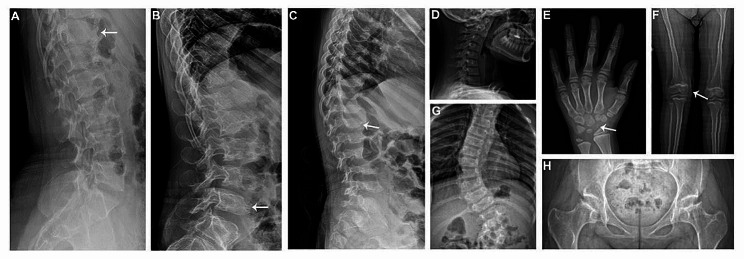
Radiographic features in mild patients with MPS IVA. Lateral lumbar spine radiographs showed irregular vertebral bodies with “tongue-shaped” deformity in Pt1 (A), anterior beaking of the vertebral bodies in Pt8 (B) and anterior sharpening of vertebral bodies in Pt9 (C). Radiographs revealed kyphotic cervical curve in Pt6 (D), dysplastic carpal bones (E) and right genu valgum (F) in Pt5, scoliosis in Pt11 (G) and acetabular and femoral head dysplasia in Pt12 (H)
